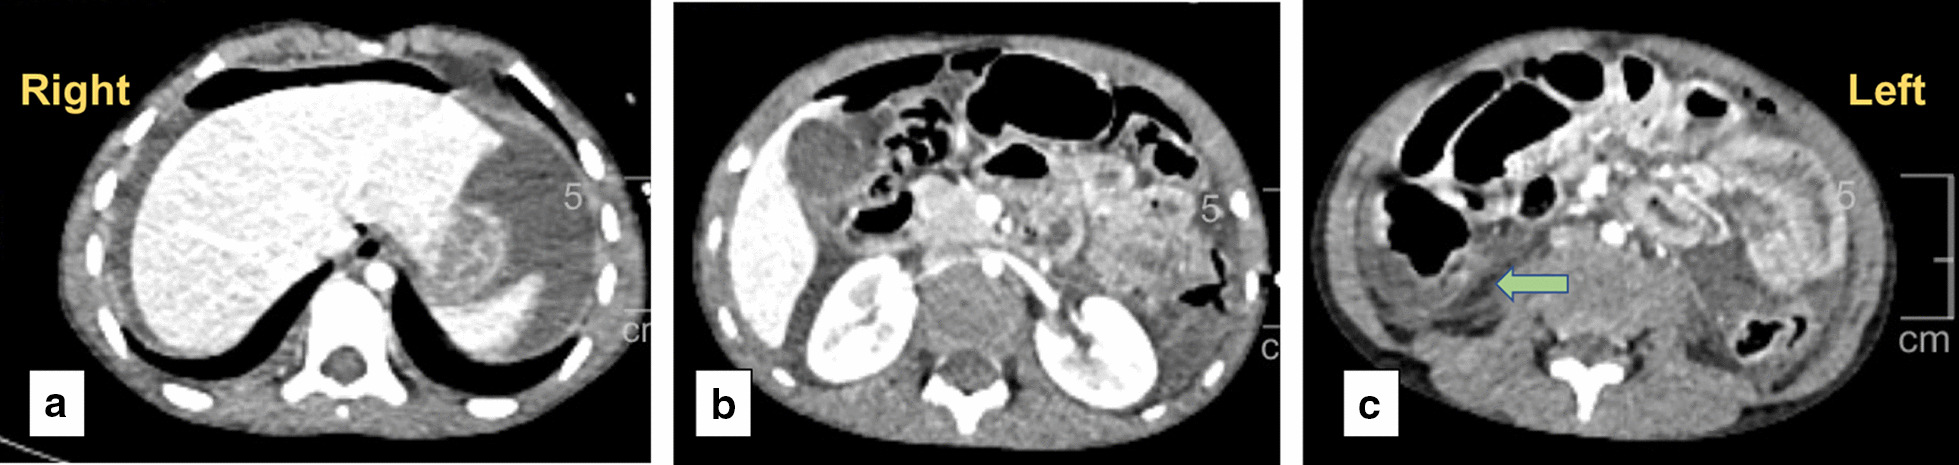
Contrast-enhanced computed tomograph (CECT) of the torso done in the emergency department showing the gross pneumoperitoneum (a) and inter-loop free air (b). The intact appendix in the right iliac fossa is identified and marked with an arrow (c).
